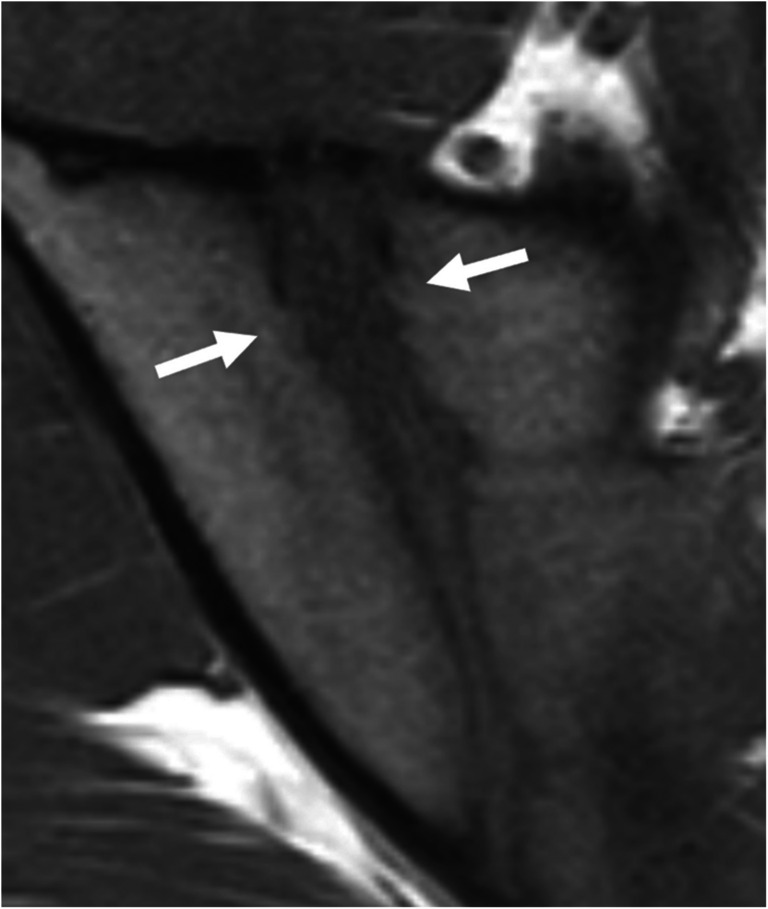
A 17-year-old man with irregularities of the SIJ mimicking erosions on coronal oblique T1-weighted image
